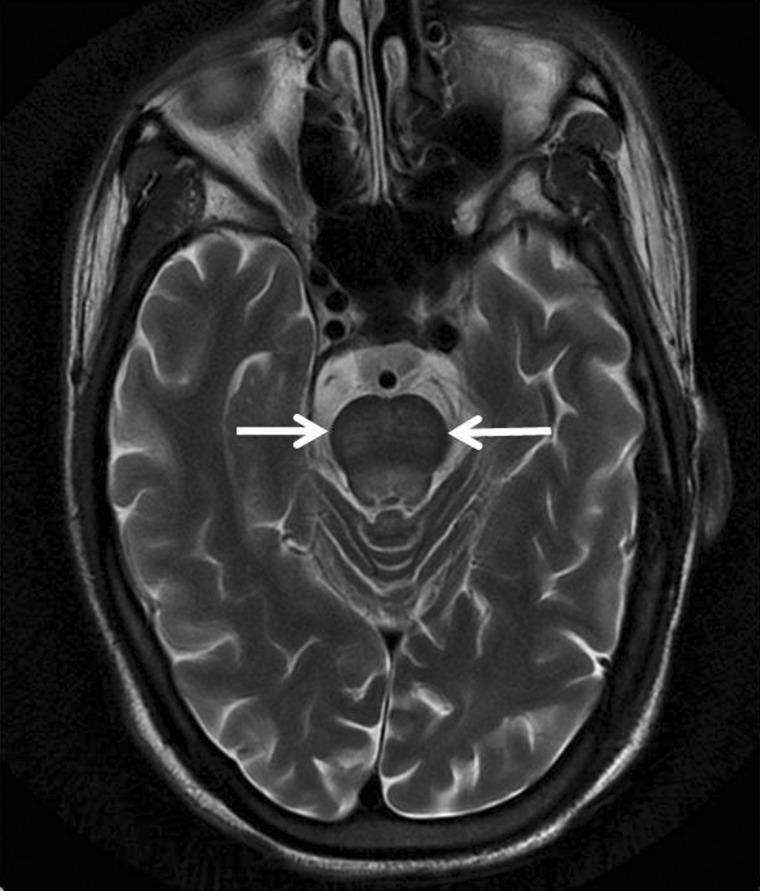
Central pontine myelinolysis. Axial T2-weighted MR brain showing central hyperintensity in pons in a case of pontine myelinolysis.
